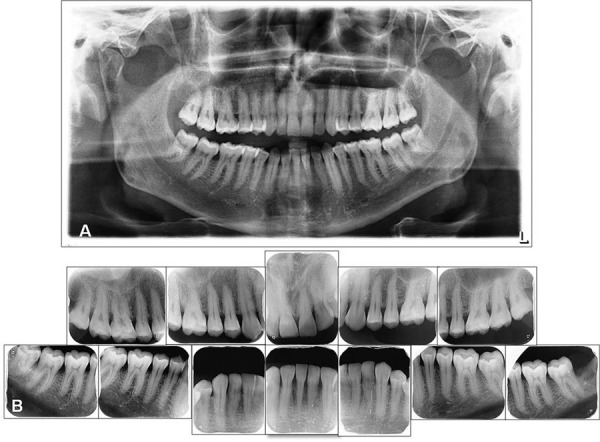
A) Panoramic radiograph of a patient with chronic periodontitis (nO-CP); B) Full mouth periapical radiographs of a patient with chronic periodontitis (nO-CP)
